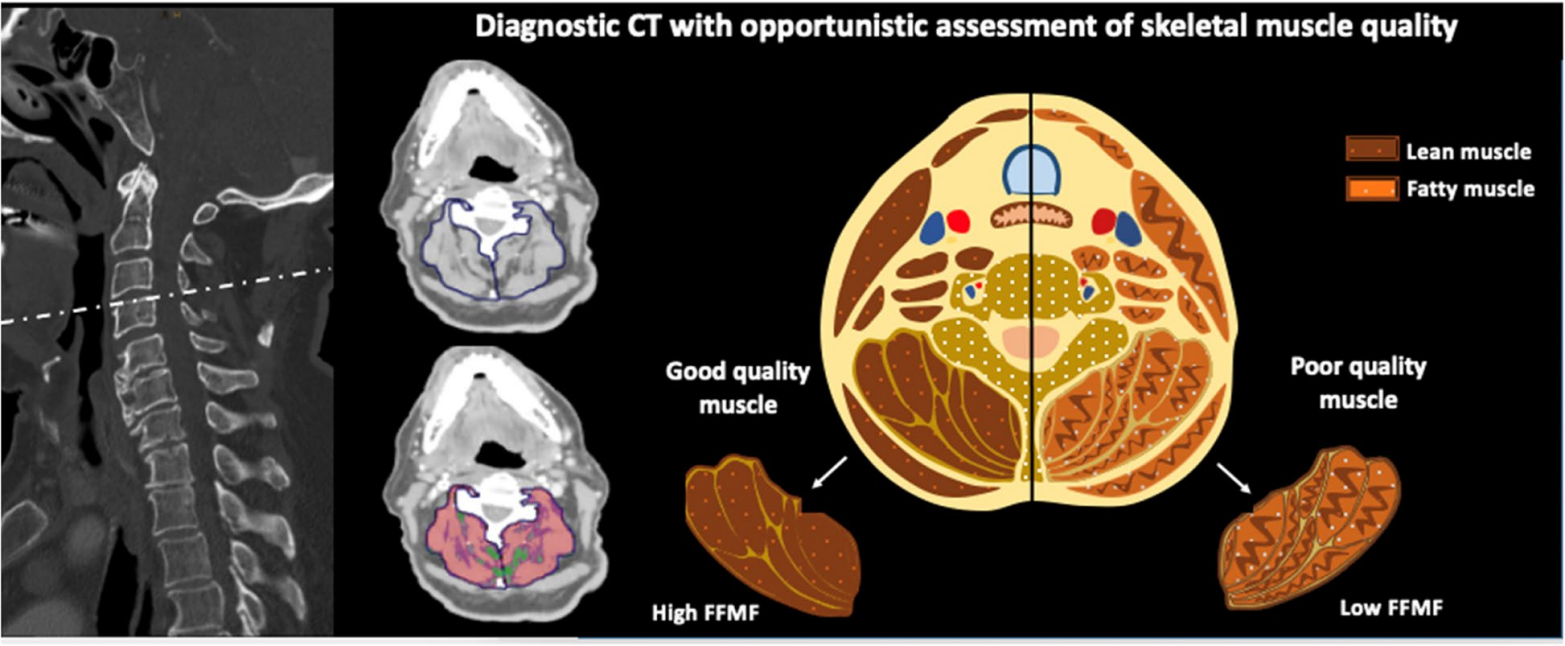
Representative images with summary of skeletal muscle composition analysis. The paraspinal muscle area at the level of the intervertebral disc space C3/4 was separated into areas of lean and fatty muscle based on densitometric thresholds. Fat-free muscle fraction (FFMF) was calculated as a relation of fatty muscle area to the total skeletal muscle area. Opportunistic analysis of CT scans was performed using an in-house software written in MATLAB. CT: Computed tomography; FFMF: Fat-free muscle fraction
